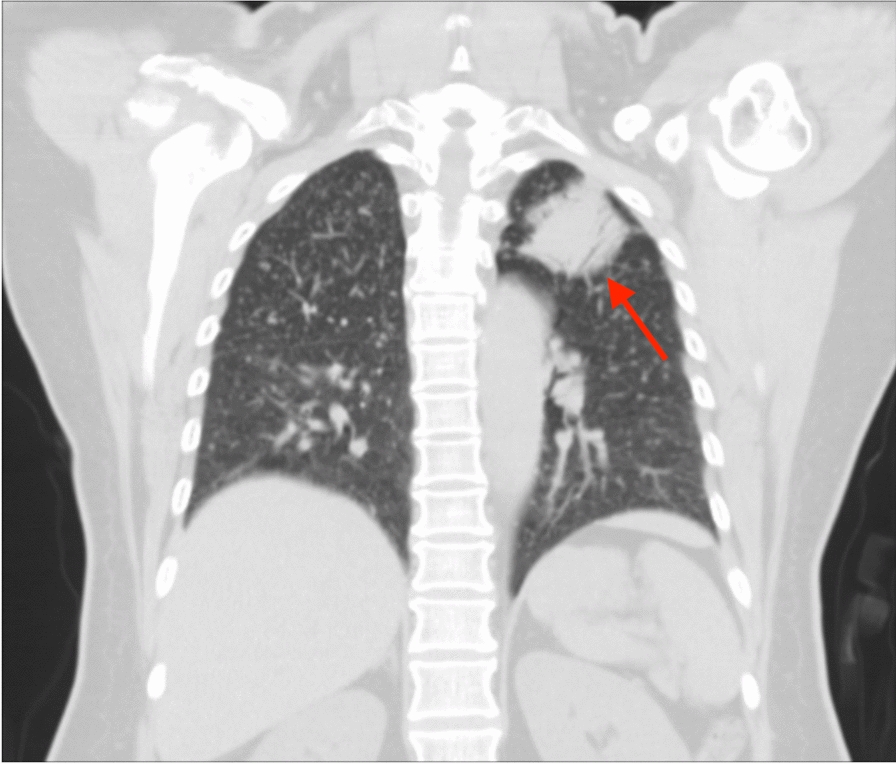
CT of the chest in lung window demonstrated a 4.4 cm mass (arrow) located at LUL of lung
